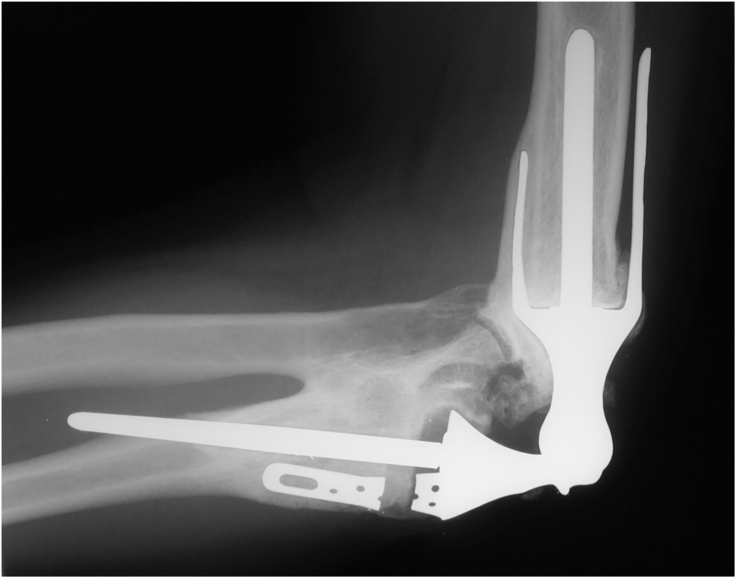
Radiograph of the original custom-made total elbow arthroplasty implanted in 1970. The humeral component exhibits both anterior and posterior flanges, and the ulnar component shows a fractured flange. The ulnar stem is positioned eccentrically, with the shaft component tracking extraosseously along the cortex. Only this archival lateral projection was available; an anterior-posterior view from the initial treatment period could not be retrieved.
